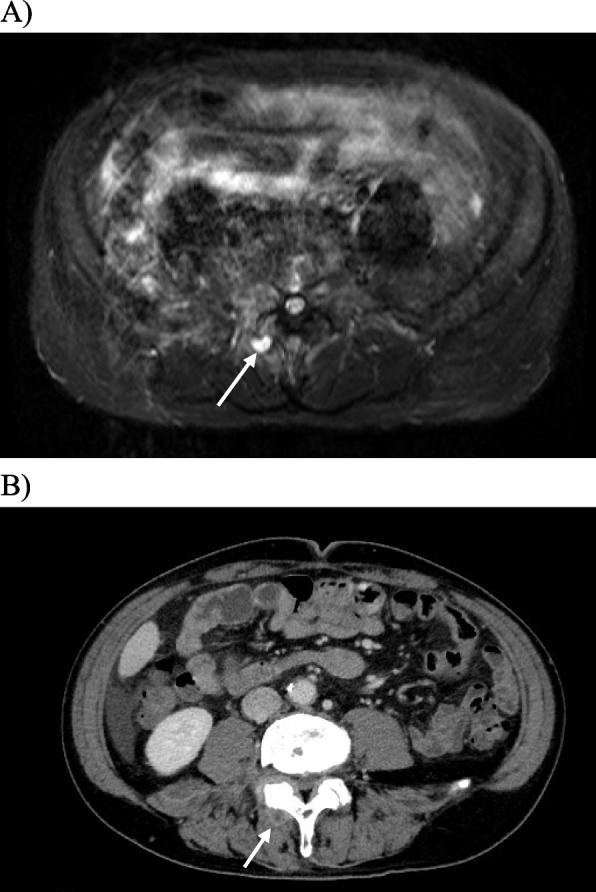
Image findings. A MRI showed high signal intensity areas in the right iliopsoas muscle and L4/L5 facet joint under STIR conditions, suggestive of fluid accumulation, indicative of inflammation, possibly pointing to disseminated lesions. B Computed tomography (CT) image at the same level
